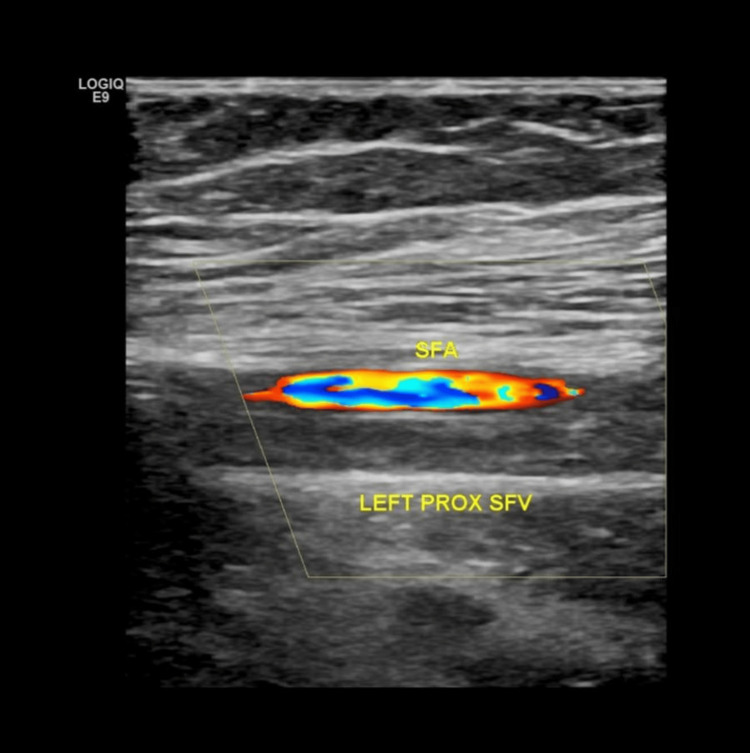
Pre-treatment color Doppler of the left superficial femoral vein
